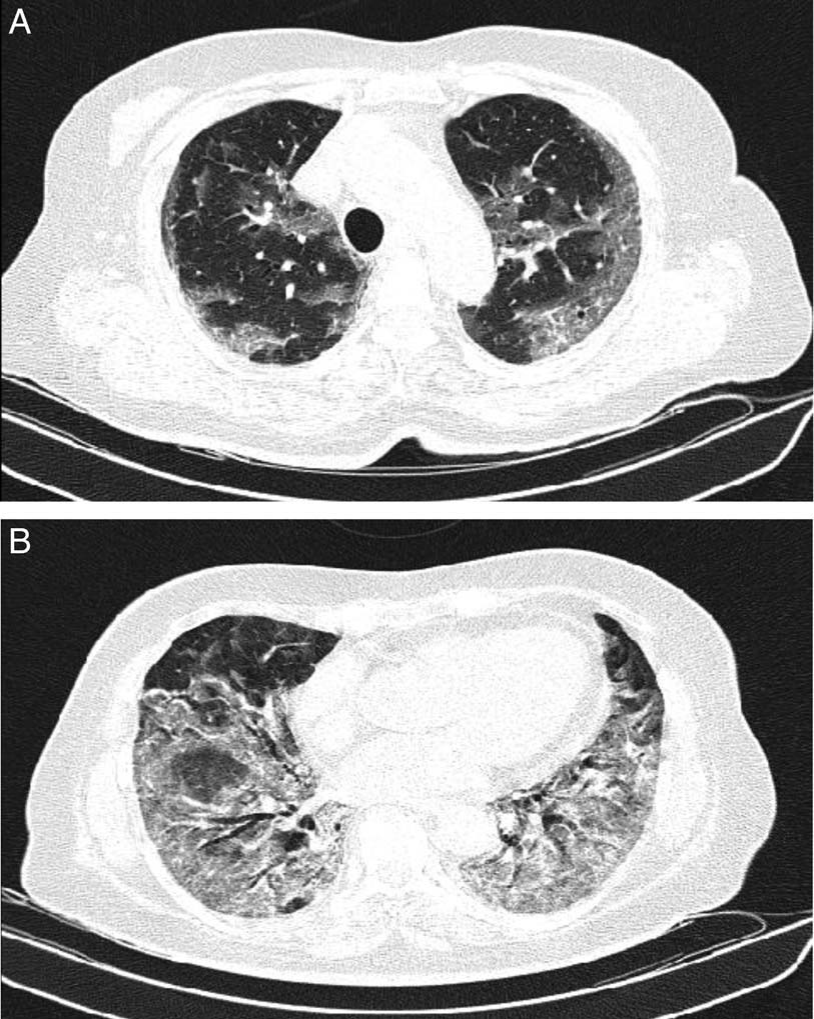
Two axial sections of the chest computed tomography scan: bilateral peripheral ground glass opacities in upper lobes (A) and diffuse consolidative and ground glass opacities in lower lobes (B).
